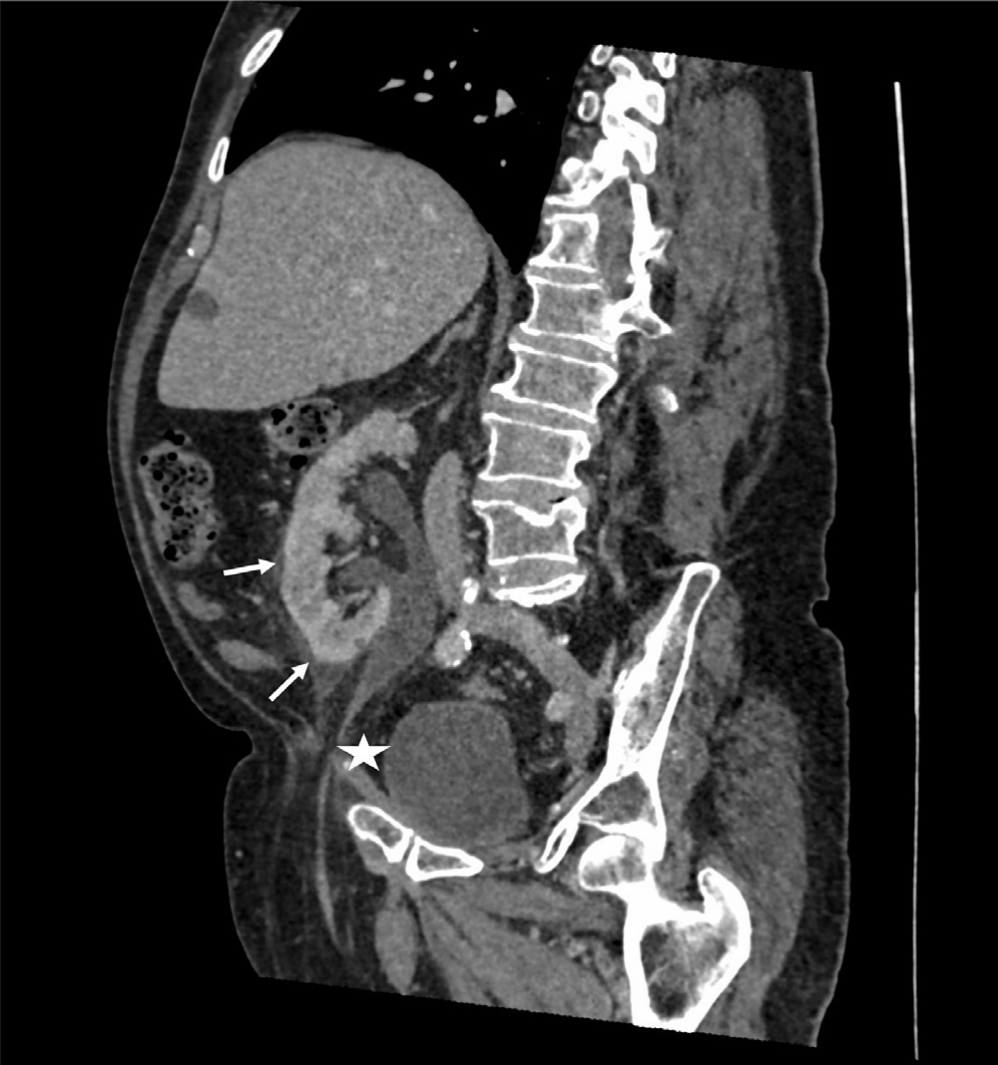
CT with coronal oblique reformat demonstrating hydronephrosis/hydroureter with a tapering point at the deep inguinal ring (white star). The kidney is hypoenhancing and there is perinephric fat stranding (white arrows).
